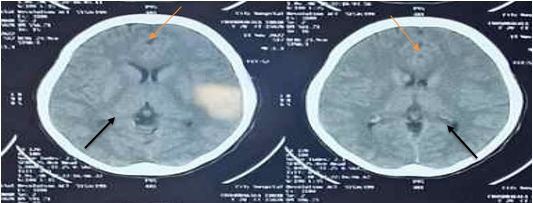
Non-contrast computed tomography showing the features of hydrocephalus (Size of both temporal horns is greater than 2 cm) of the ventricles (Black Arrow) and bilateral temporal showing cerebral infarct (Orange Arrow).
